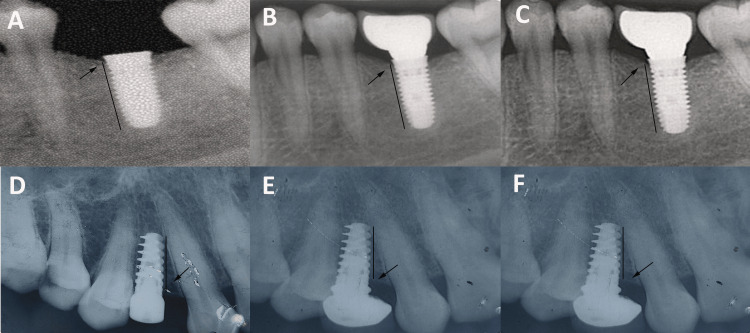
Crestal bone height: (A) at baseline in flapless surgery, (B) at the sixth month in flapless surgery, (C) at the 12th month in flapless surgery, (D) at baseline in flap surgery, (E) at the sixth month in flap surgery, and (F) at the 12th month in flap surgery. This figure represents radiographic images from patients who participated in this study. All identifying information has been removed to maintain confidentiality.
